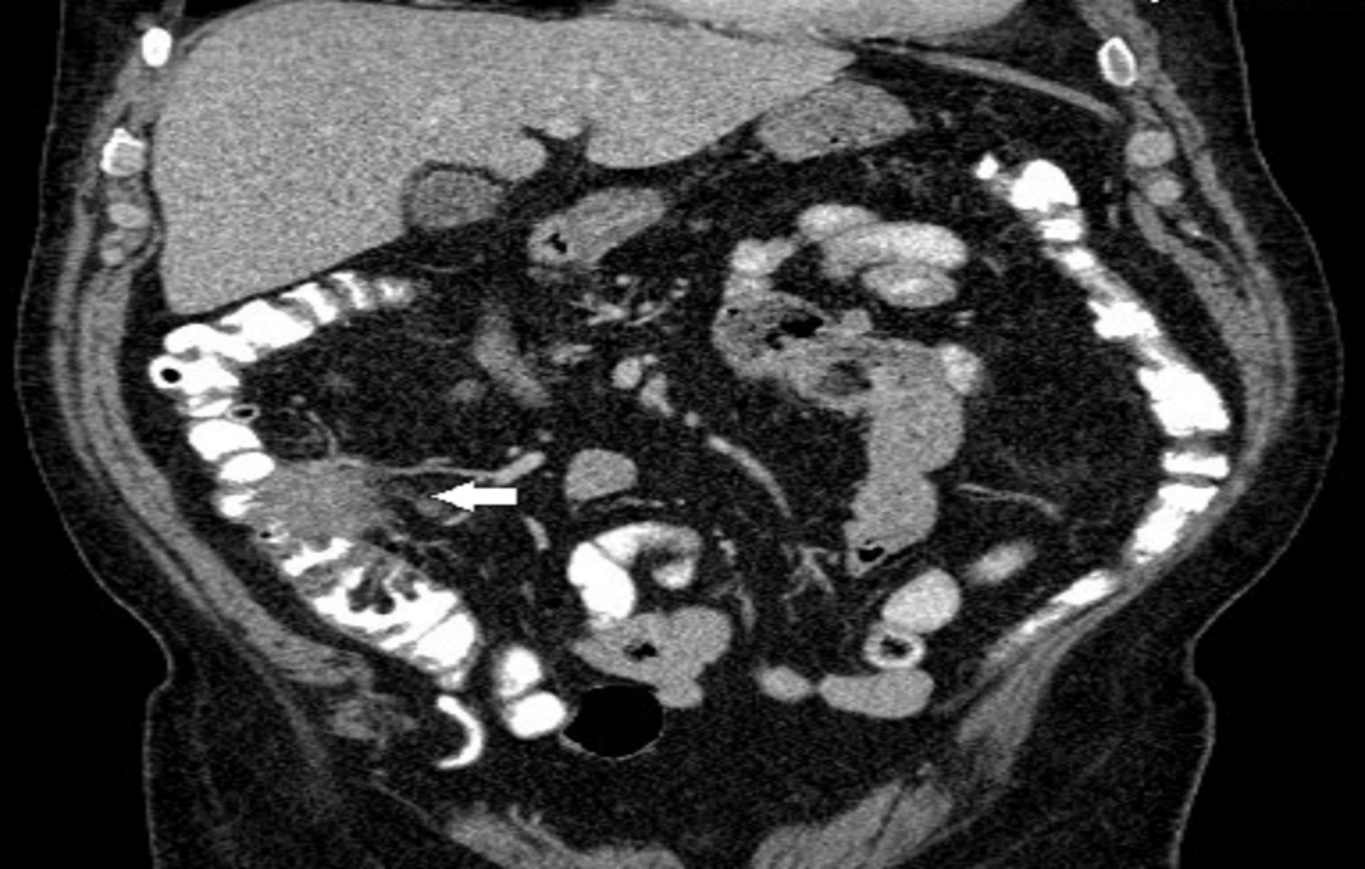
Abdominal computed tomography scan, coronal view, showing an irregular 5.5 cm soft tissue mass abutting the right colon medially
